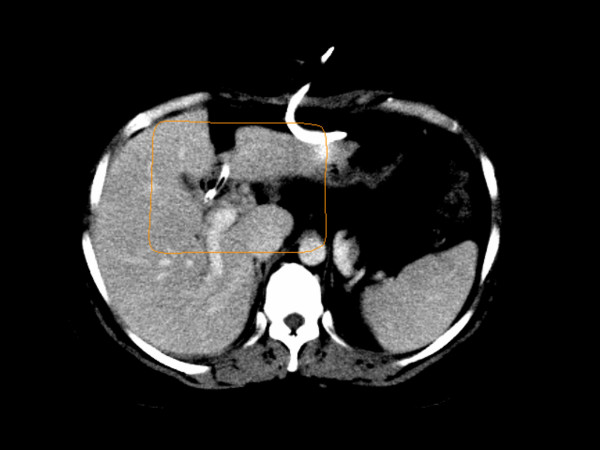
Axial image of CT scan performed with IV contrast for radiation treatment planning. The solid line marks the region that received at least 50.4 Gy during the radiotherapy course.
